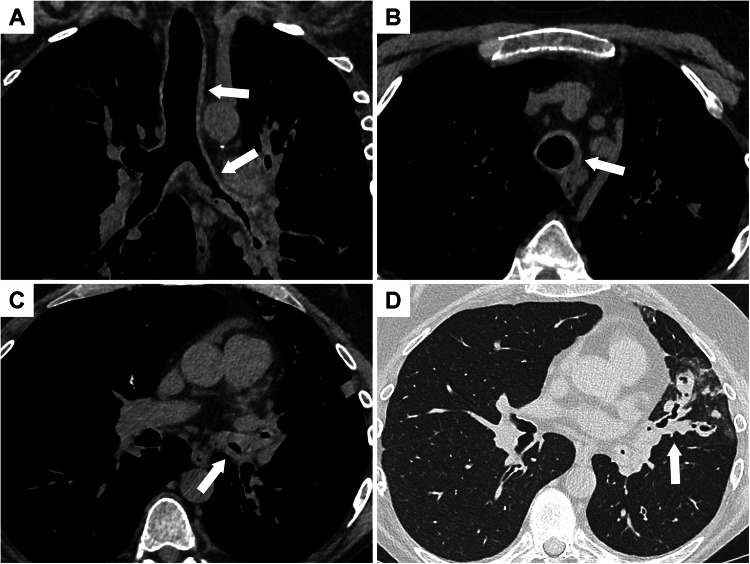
Chest CT showing airway involvement in a 48-year-old patient with ulcerative colitis diagnosed 25 years before. A CT image in the coronal plane shows tracheal and bronchial wall thickening (arrows). Axial images show tracheal wall thickening sparing the posterior membrane (B) and bronchial wall thickening of the left lower lobe bronchus with stenosis (C). D These lesions are associated with bronchial dilatation and mucus impactions (arrow) in the left upper lobe
